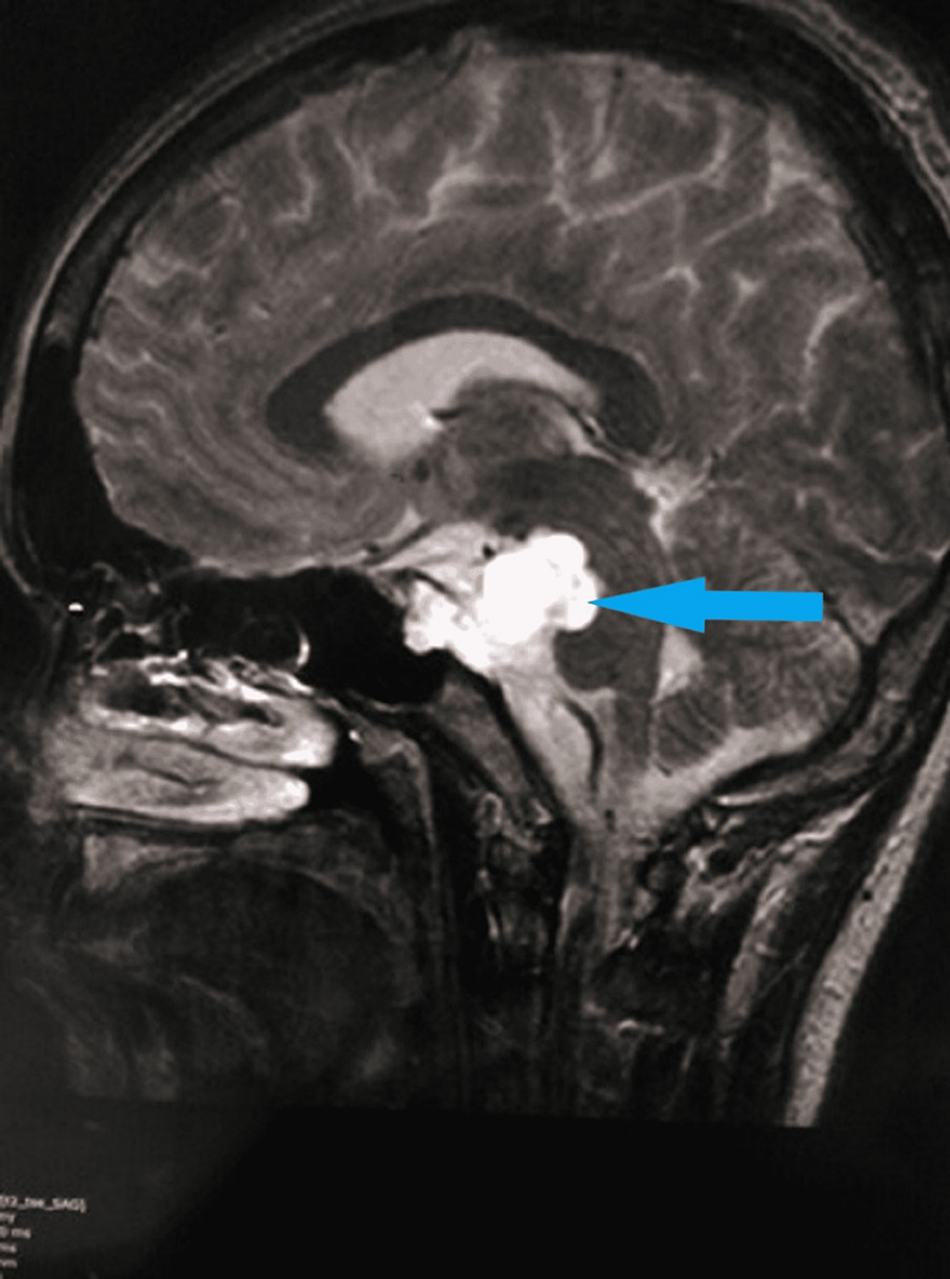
T2 sagittal MRI scan of the brain shows a hyperintense lesion in the retroclival region without any bony change. MRI: magnetic resonance imaging
